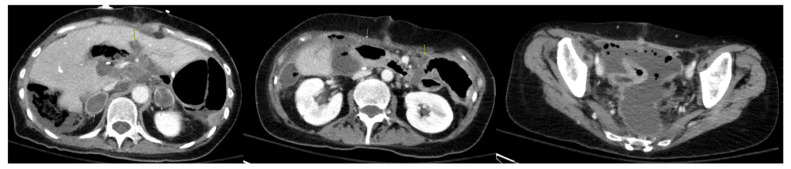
CT of the abdomen/pelvis after second CRS/HIPEC. Arrows indicate sites of residual postoperative collections.
